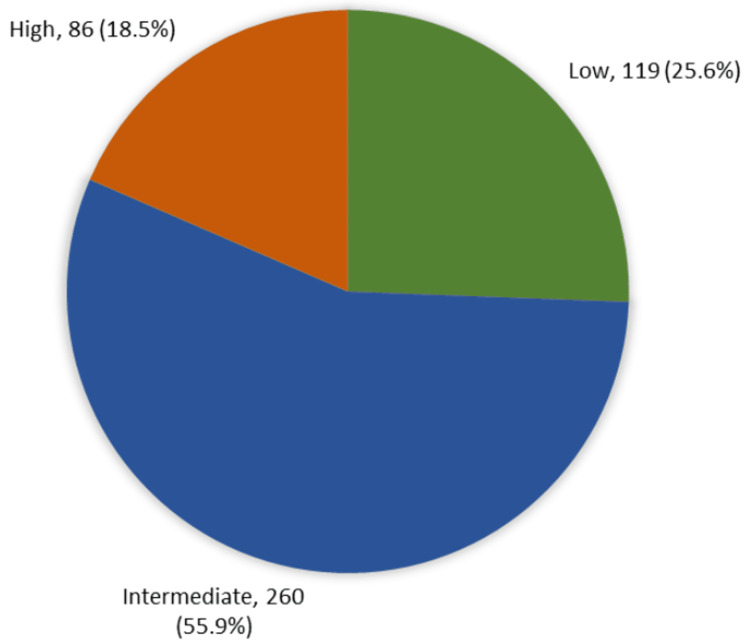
Overall anxiety level associated with MRI examination among the general population of the western region in Saudi Arabia MRI: magnetic resonance imaging
